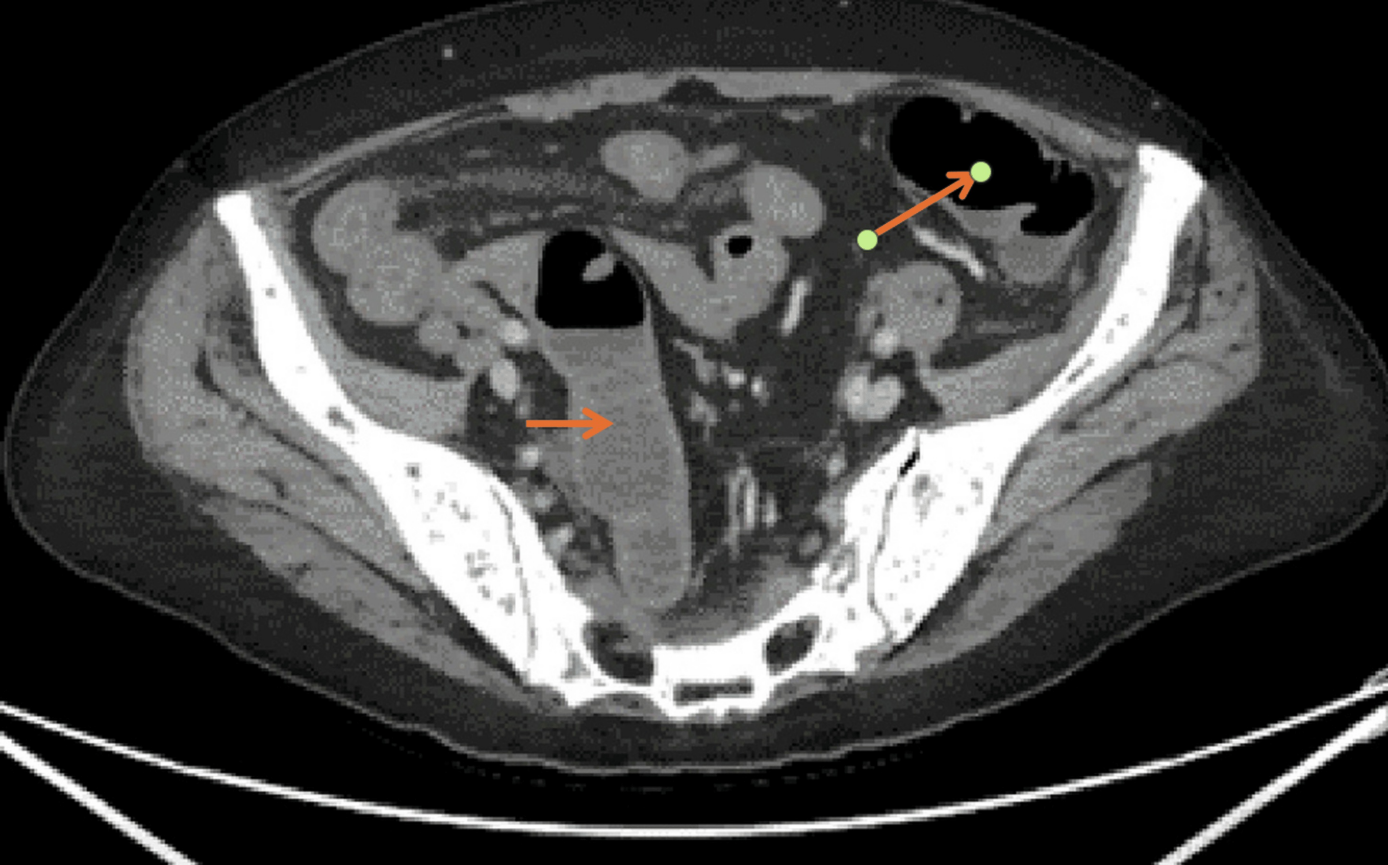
CT abdomen showing trace amounts of free pelvic fluid without evidence of drainable collections (arrows).
